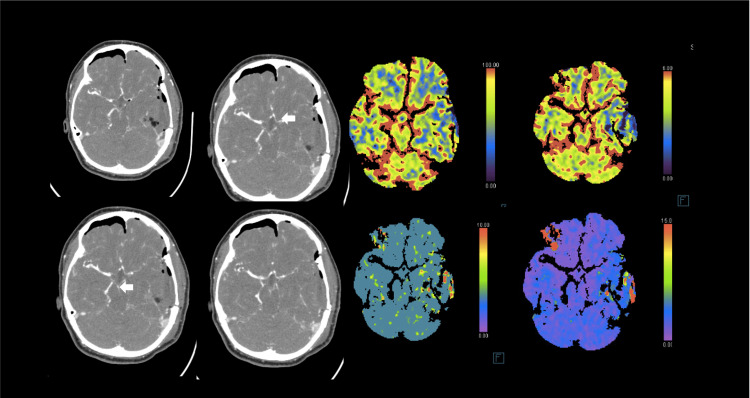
Postoperative imaging CTA demonstrating vasospasm involving the basilar artery and left internal cerebral and middle cerebral arteries that were not evident in the preoperative CTA. CT perfusion showed no evidence of core infarction or ischemic penumbra. CTA: computed tomography angiography
